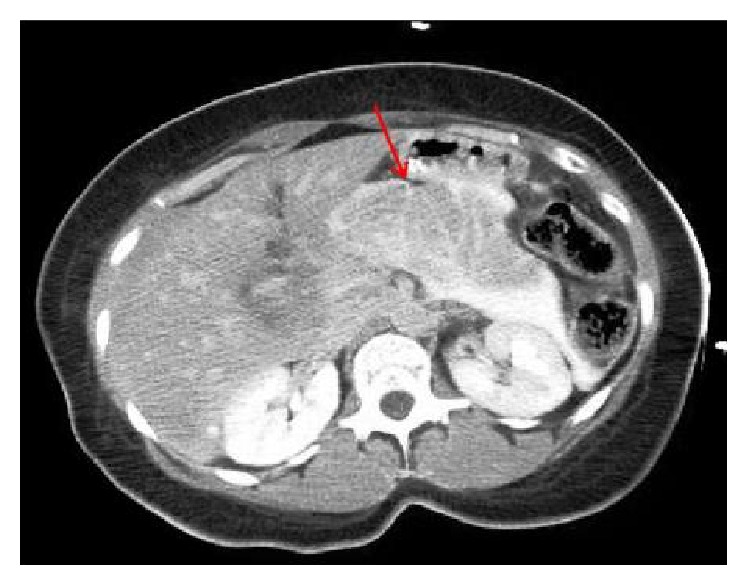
CT abdomen with contrast image showing swollen inflamed, edematous pancreas.
